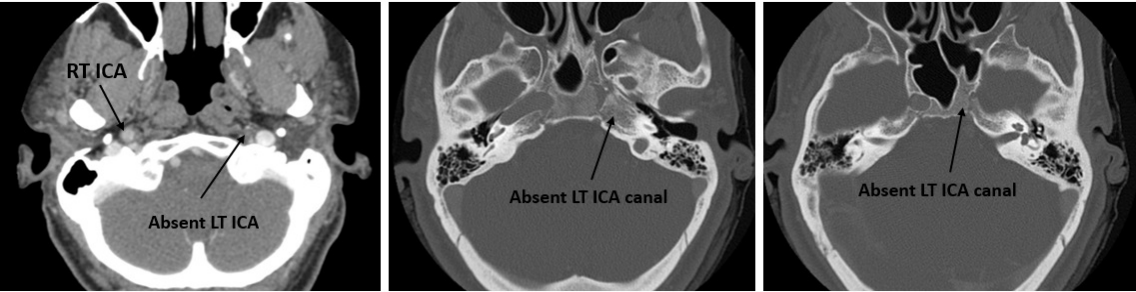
Computed tomography (CT) scan images of the skull base; the absence of left internal carotid artery (ICA) and corresponding bony canal is visible
